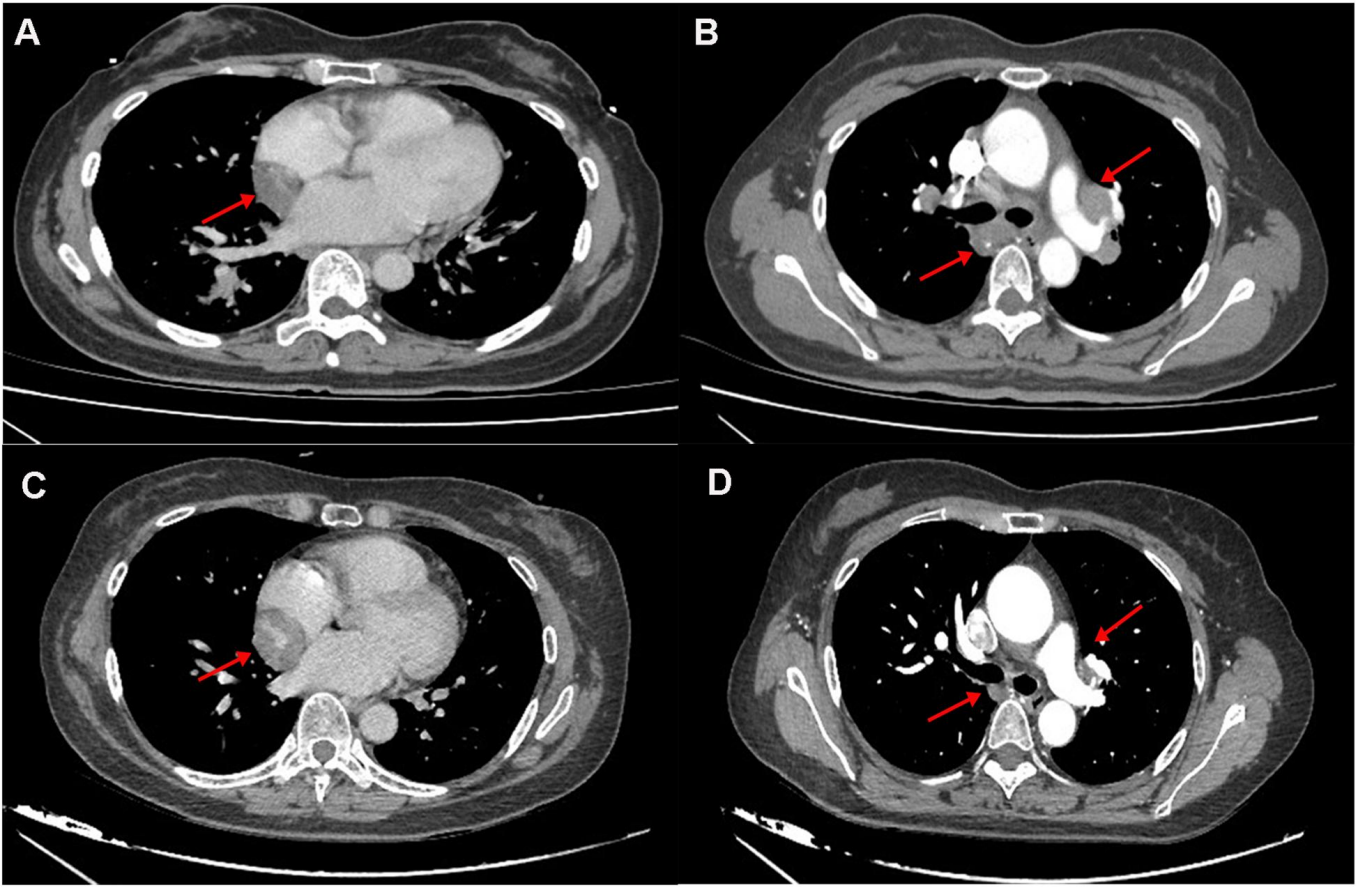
(A,B) the 2022 chest CT revealed a hypodense lesion in the interventricular groove and hilar lymphadenopathy. (C,D) The 2025 CT revealed progressive enlargement of a mass within the atrioventricular groove (measuring 3.3 cm vs. 4.1 cm in 2022), with concurrent regression of bilateral hilar lymphadenopathy.
